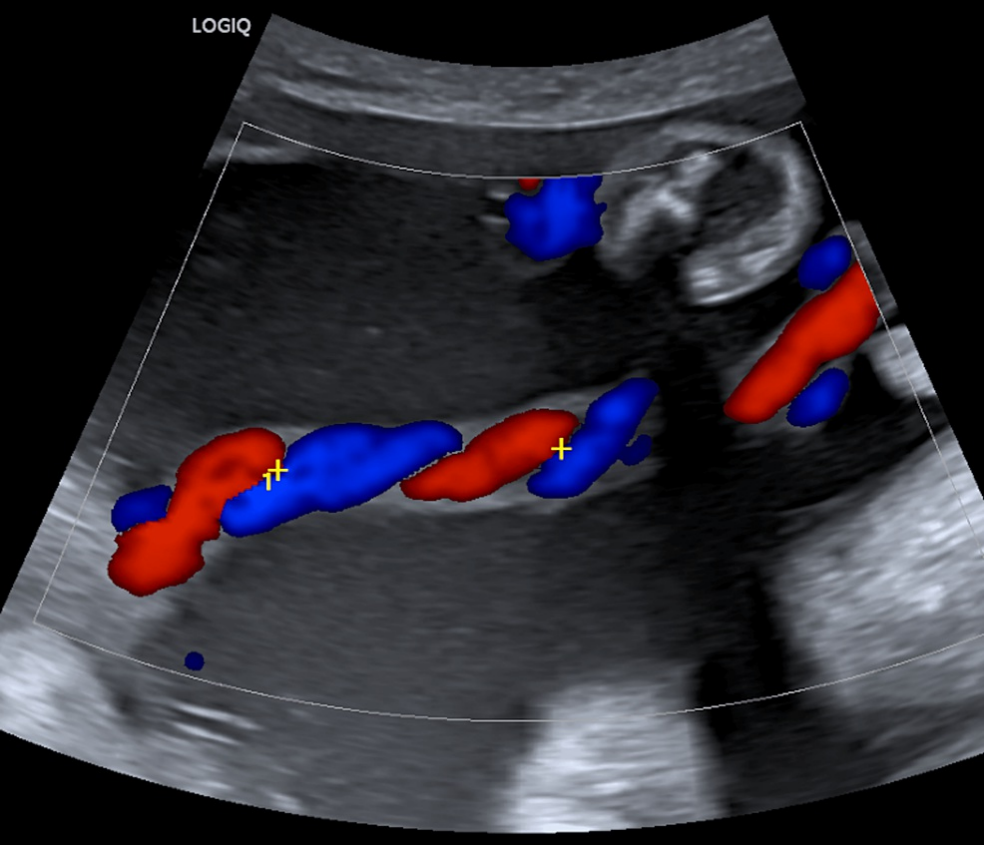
Color doppler USG image showing a free loop of umbilical cord. One coil containing 2 arteries and one vein depicted within the calipers.
